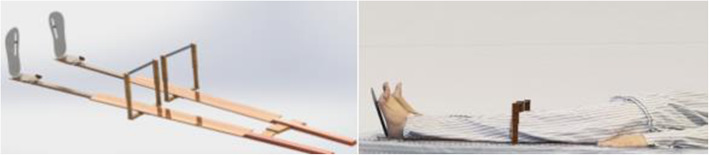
A specialized patented device designed to ensure the same posture fixation while X-ray and CT were performed
